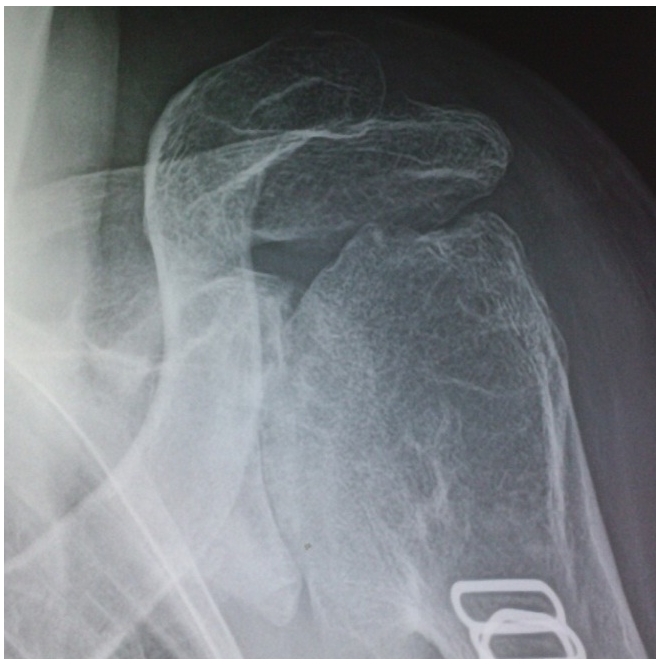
Radiographie de l’épaule de la patiente atteinte d'acromégalie montrant une arthropathie destructrice
